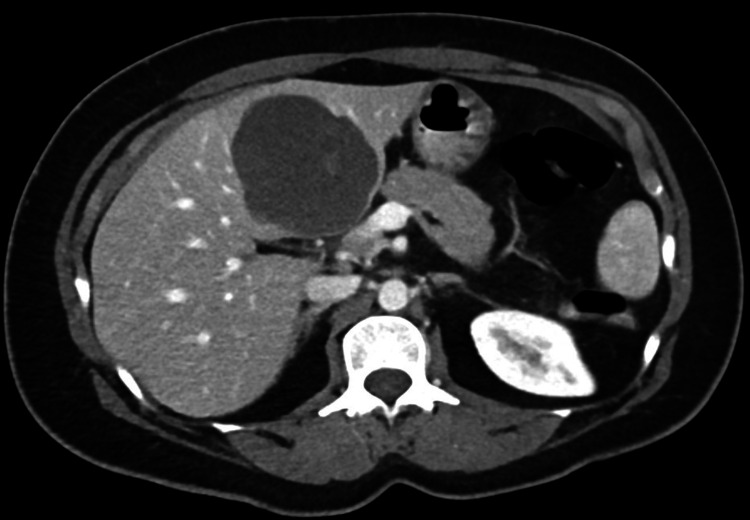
Post-contrast (venous phase) CT scan of the liver showing a complex cystic mass lesion in the left lobe of the liver with an enhancement of the wall and the septae
